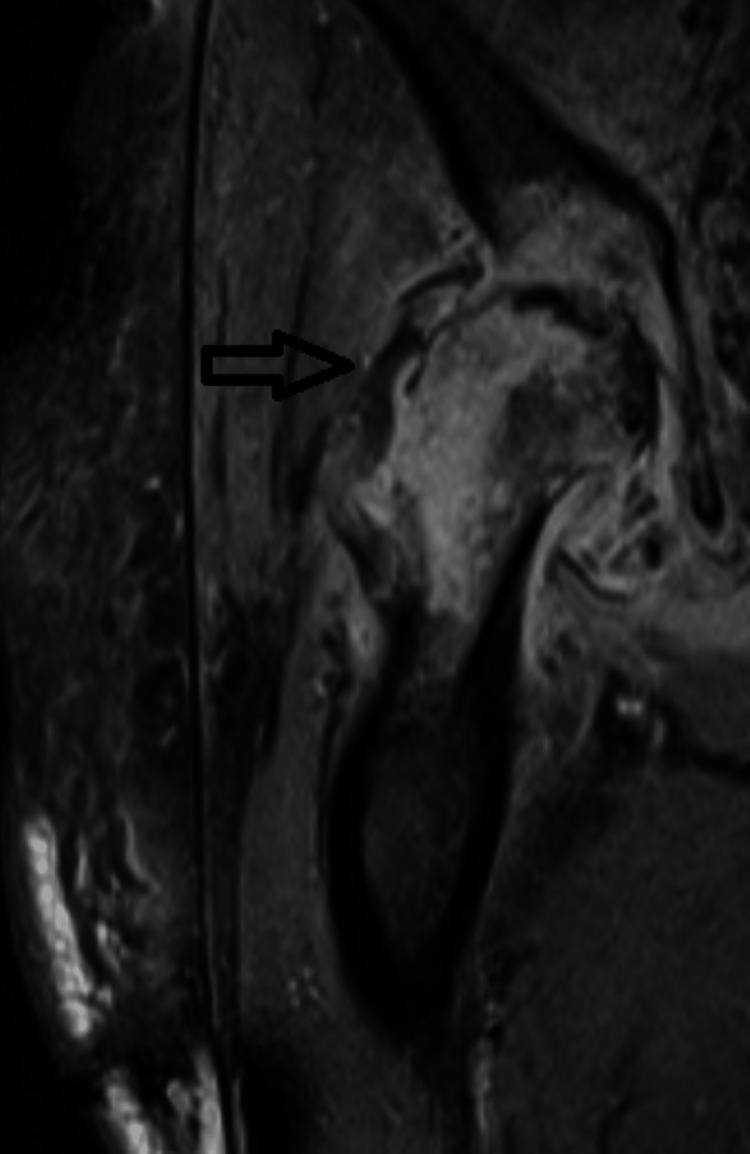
MRI T1-weighted fat saturation post-contrast coronal image of right hip showing sequelae of septic arthritis and extensive osteomyelitis (arrow).
